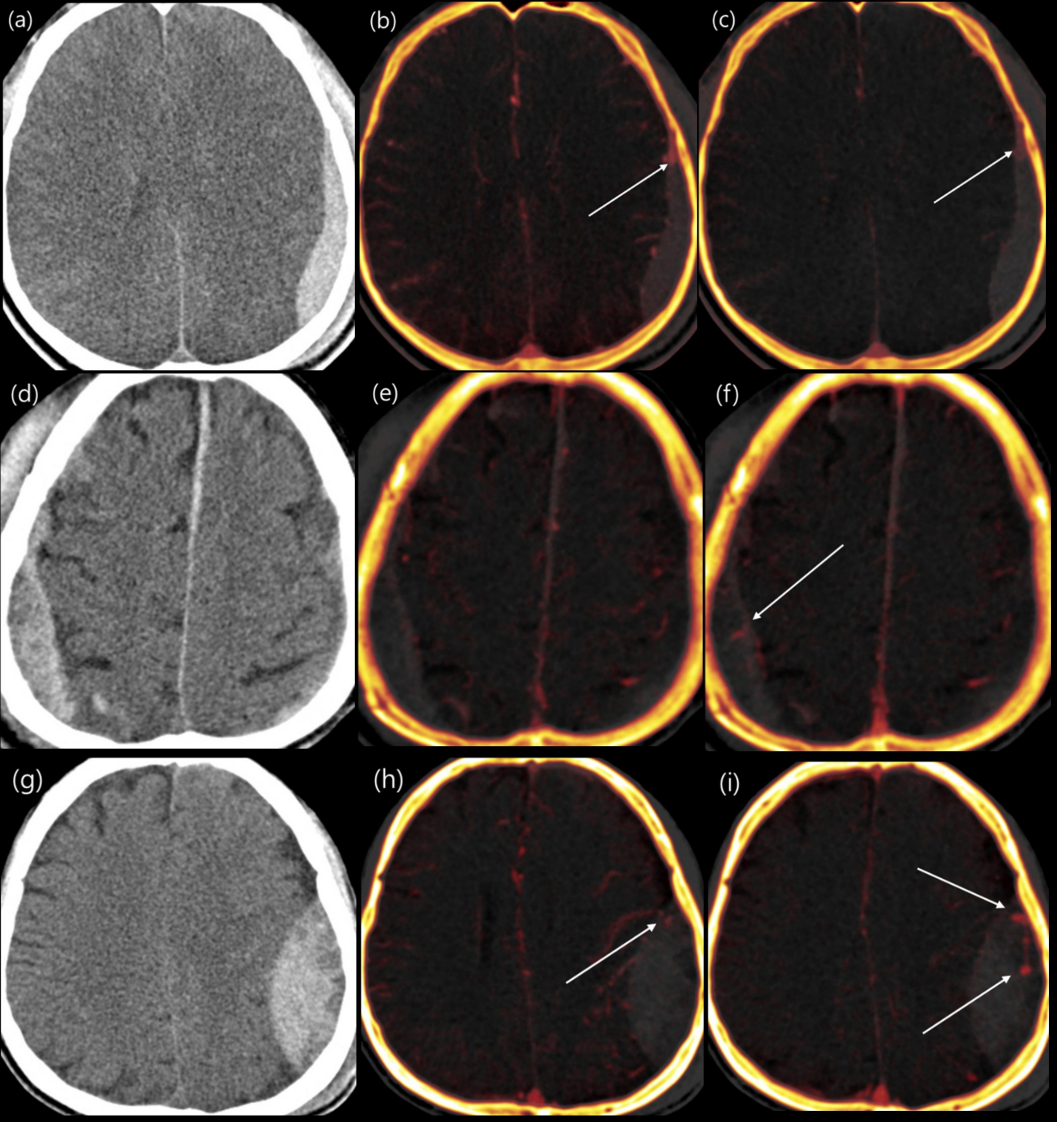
Classification of contrast leakage patterns. Dual-energy CT images (conventional-like images: a, d, g; arterial phase fusion images: b, e, h; venous phase fusion images: c, f, i) showing three contrast enhancement patterns: Intralesional enhancement without change (a–c), delayed enhancement (d–f), and growing contrast leakage (g–i).
